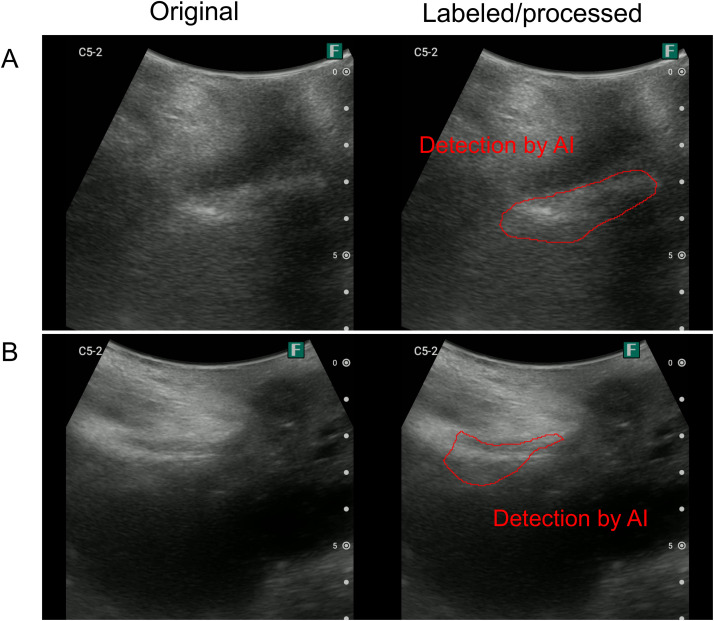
Examples of ultrasound images determined as false positives. (Left) Original image. (Right) Areas labeled as correct by the sonographer (yellow) and detected by AI (red). Despite the sonographer’s determination of no hyperechoic findings indicating fecal retention, the AI misidentified the images as false negatives. AI, artificial intelligence; IoU, intersection over union.
